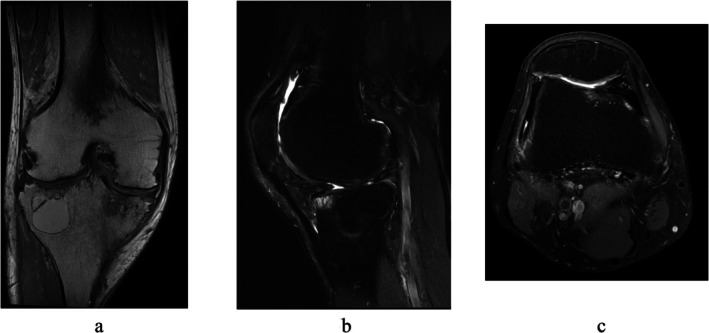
MRI before the second look surgery. (a) Proton density‐weighted image (coronal section). (b) Proton density fat‐suppressed image (sagittal section). (c) Proton density fat‐suppressed image (horizontal section).
